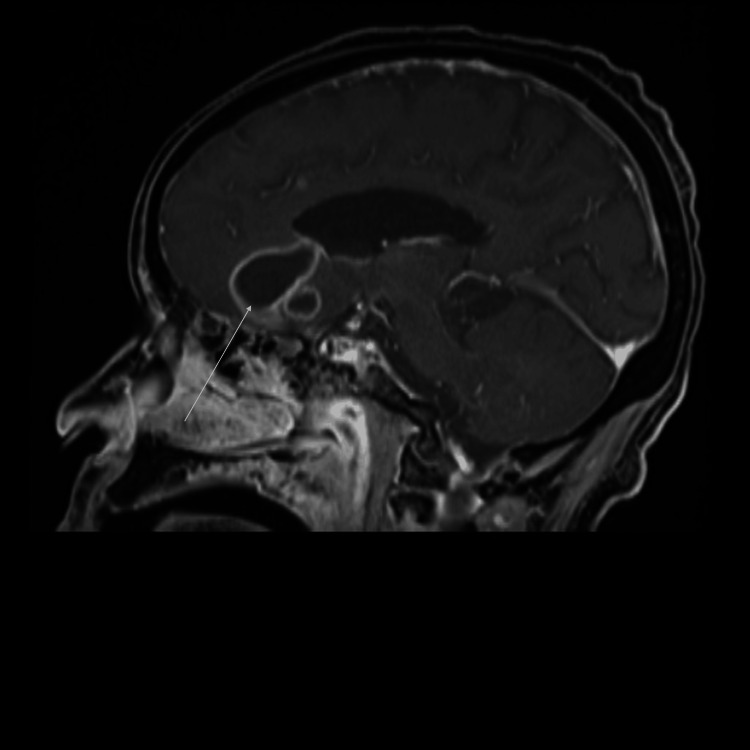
Initial brain MRI showing vasogenic edema in the inferior aspect.
